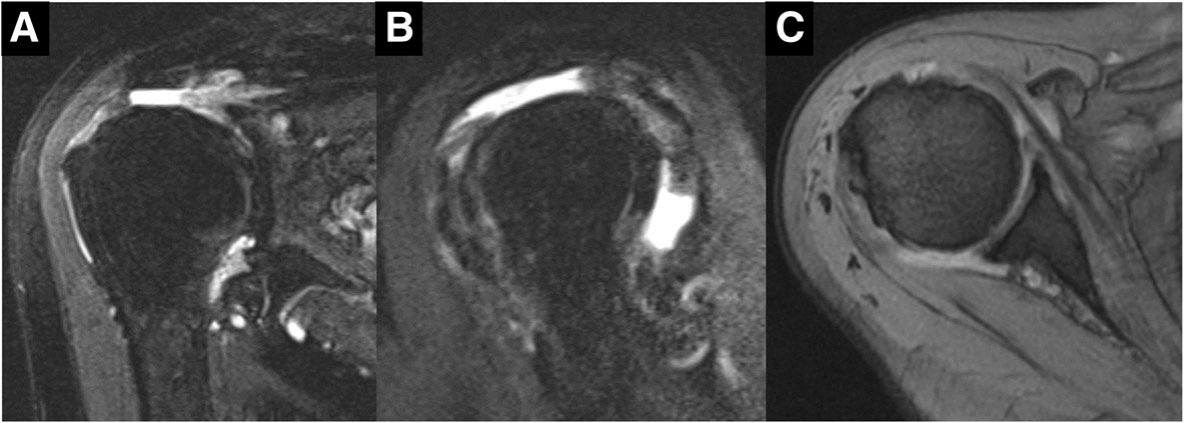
MRI images of the right shoulder 2 years before the trauma. Fat-suppressed T2-weighted oblique axial (a) and oblique sagittal (b) images, and T2-weighted oblique coronal image (c) revealing a complete tear and retraction of the supraspinatus tendon and a rupture of the long head of the biceps tendon. MRI, magnetic resonance imaging
